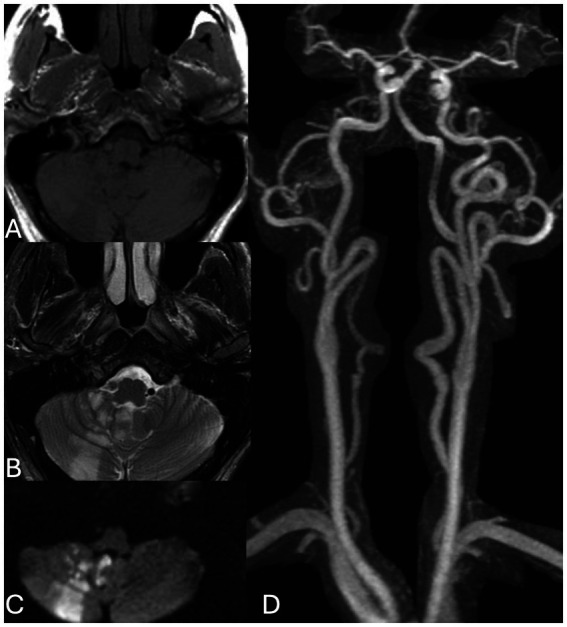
Baseline MR imaging during acute presentation: axial T1 (A), T2 (B), and DWI (C) sequences as well as a contrast enhanced MRA (D) demonstrate a missing flow void in the right vertebral artery, acute ischemia in the PICA territory and no contrast in the right V4 segment in keeping with an acute vertebral artery dissection. There is no aneurysmal dilatation at this point. Note multiple irregularities of the bilateral V2 segments as well as elongated arteriopathy in keeping with potential underlying collagenopathy.
